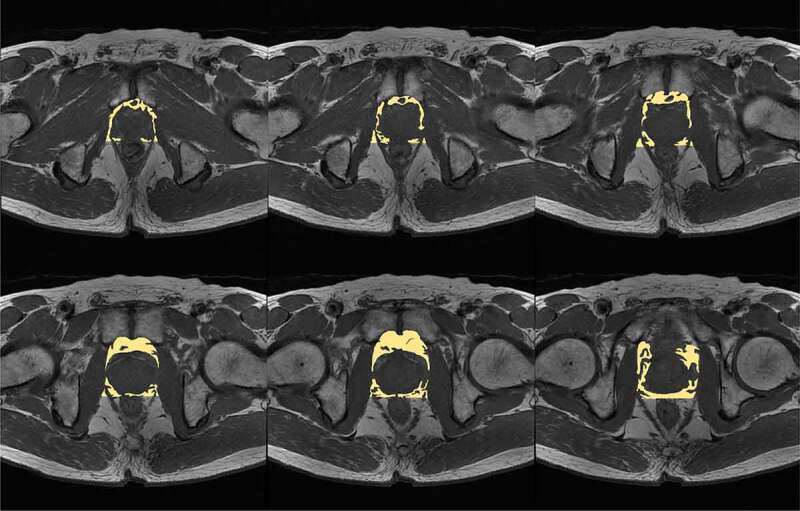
Measurement of PPAT volume on multiple T1-weighted slices. PPAT: periprostatic adipose tissue.
